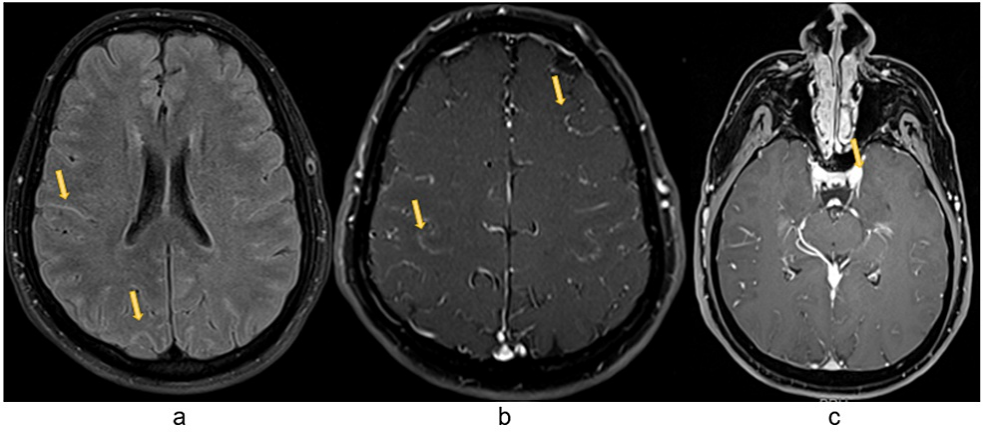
Brain MRI (FLAIR sequence) showing hyperintense signal in CSF space, especially in the sulci (arrows in panel a), diffuse pial signal gadolinium enhancement (arrows in panel b) as well as enhancement of both third cranial nerves, more intense on the left one (arrow in panel c). CSF: Cerebrospinal fluid; FLAIR: Fluid-attenuated inversion recovery.
